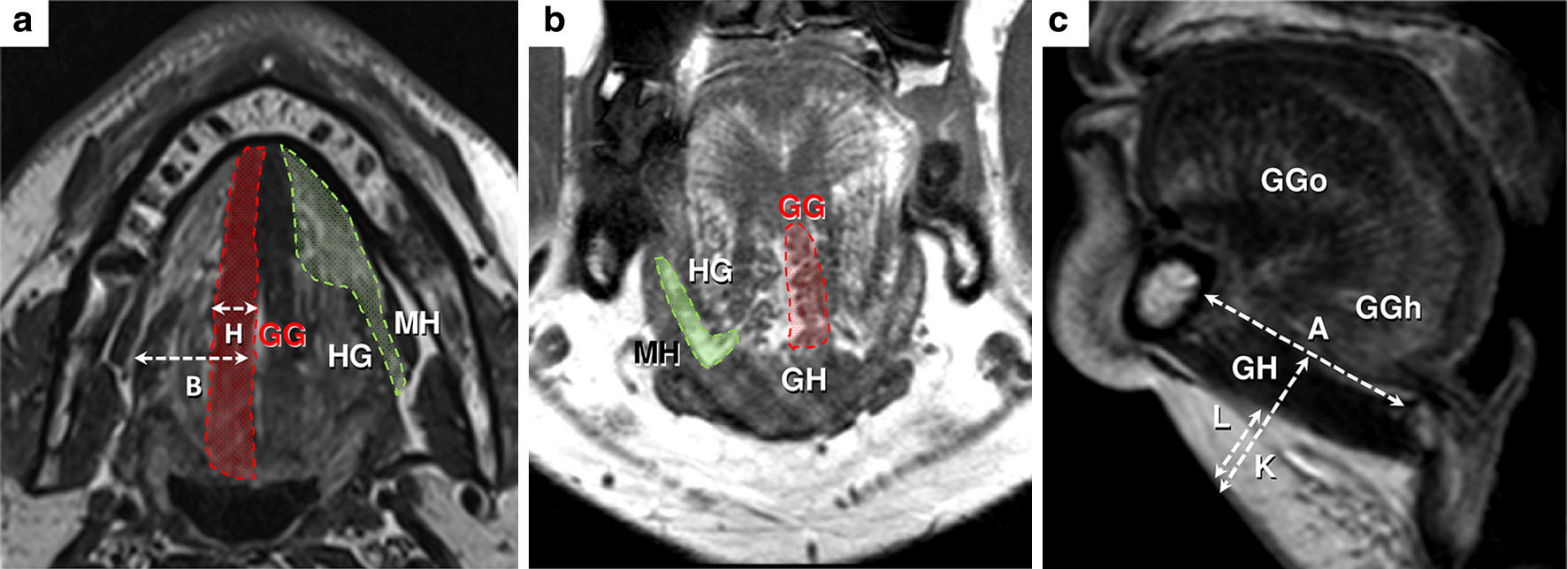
MRI study landmarks of the oral floor. a Axial section (T2-weighted). b Coronal section (T1-weighted). c Sagittal section (T2-weighted). Anatomical structures are labeled as follows: mylohyoid (*MH*), hyoglossus (*HG*), geniohyoid (*GH*), genioglossus (*GG*), horizontal fibers of GG (*GGh*), oblique fibers of GG (*GGo*). A single genioglossus area is highlighted in *red*. Sublingual regions are highlighted in *green*. Measurements were realized as indicated by *white arrows*: mandible-hyoid (*A*), skin to GG muscles (*K*) and skin to GH inferior surface (*L*) distances (color figure online)
